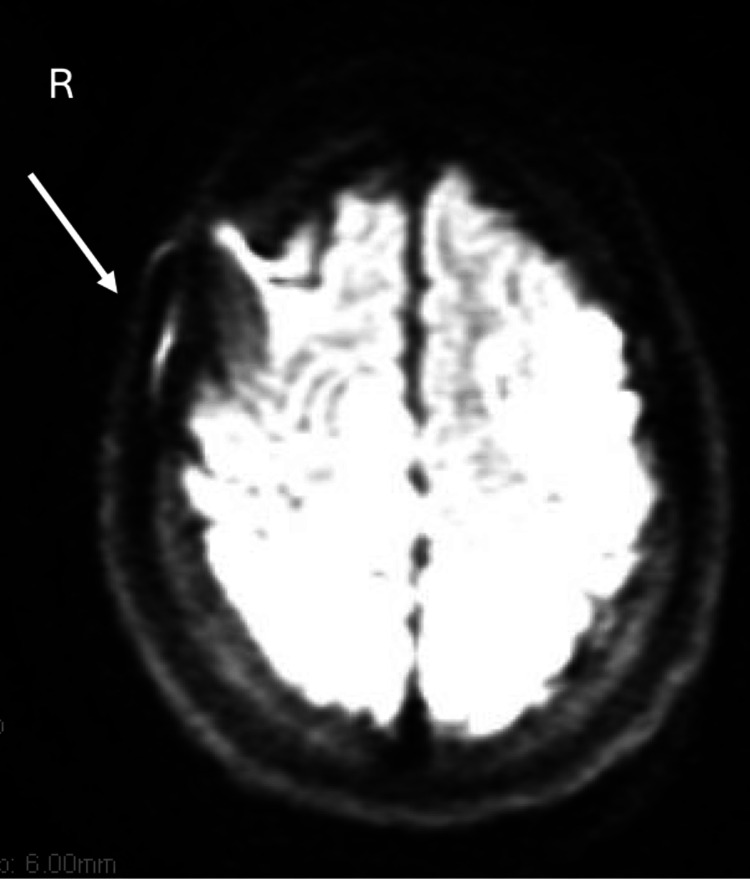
MRI of the brain demonstrating an area of acute ischemic injury (arrow) as one of several sites resulting from vascular compromise. Image quality is limited due to motion artifact.
